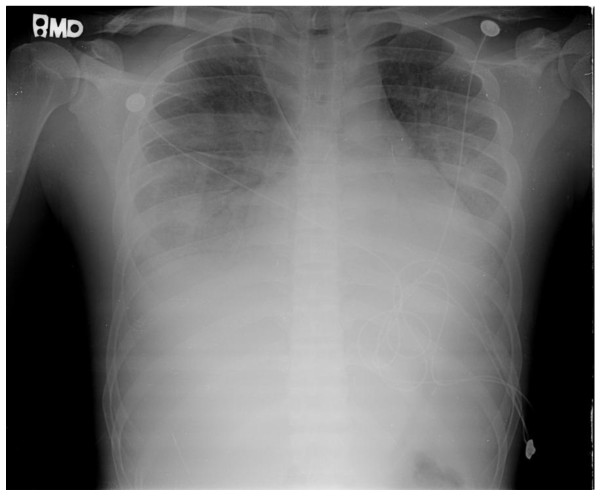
Chest x-ray revealed bilateral diffuse infiltration disclosing a presentation of acute respiratory distress syndrome.
